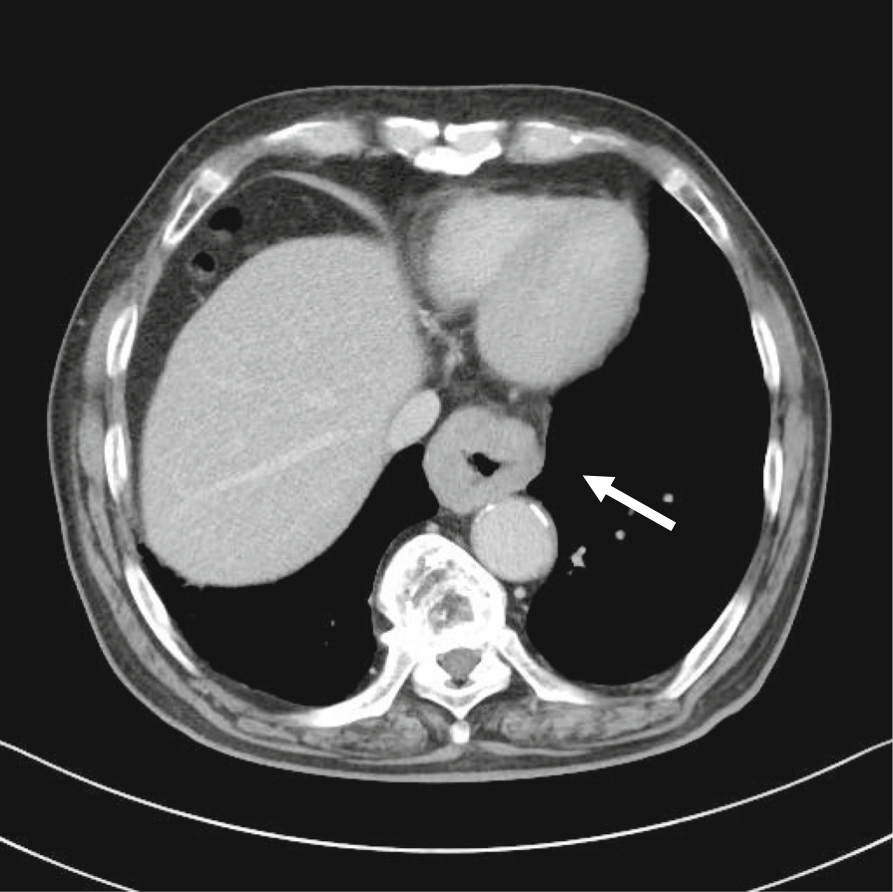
Computed tomography (CT) of the thorax revealed wall thickening from the lower thoracic esophagus to the abdominal esophagus (arrow)
